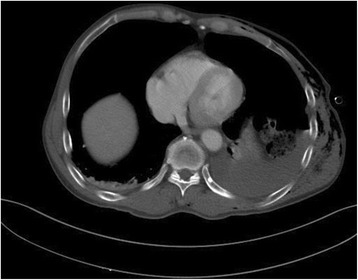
Chest computed tomography scan taken at admission revealed multiple ribs fracture and hemopneumothorax, left
